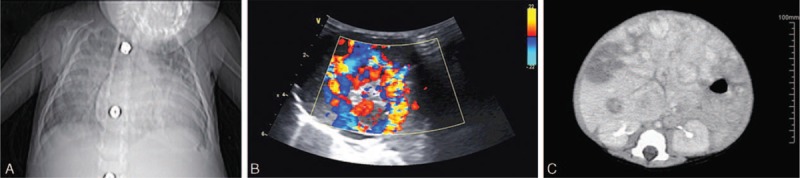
Chest radiograph, Doppler ultrasound, and computed tomography of patient 1. A, chest radiograph showing heart enlargement and a cardiothoracic ratio of 0.75. B, Doppler ultrasound showing a non-uniform echo pattern with prominent vascularity in the left lobe of the liver. C, Abdominal computed tomography with contrast revealed intrahepatic multiple nodules, or circular enhancement with irregular margins, but the central region was non-enhanced. Cystic part or low-density component around the lesion.
